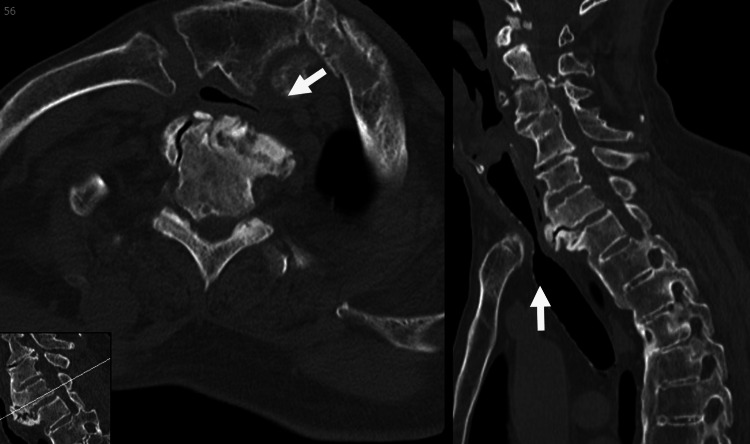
Plain CT of the upper thoracic level The arrow indicates the space-occupying lesion and tracheal stenosis at the thoracic inlet.
